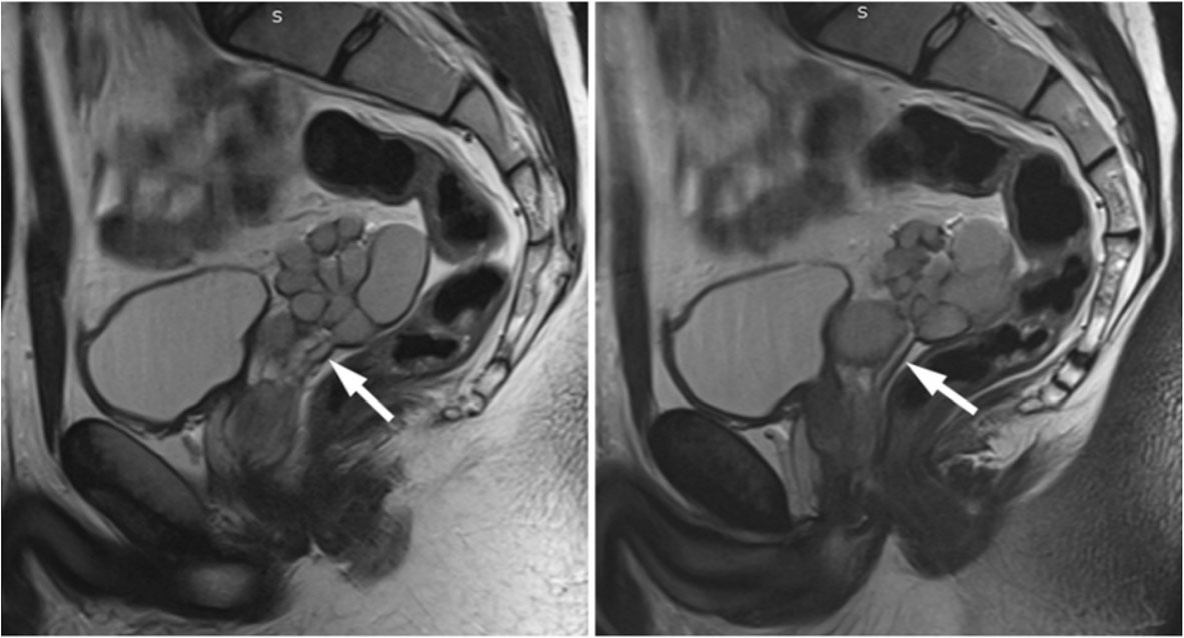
Sagittal T_2_-weighted MR images of the pelvis demonstrate enlargement of left ejaculatory duct communicating with the dilated SVC (arrows)
